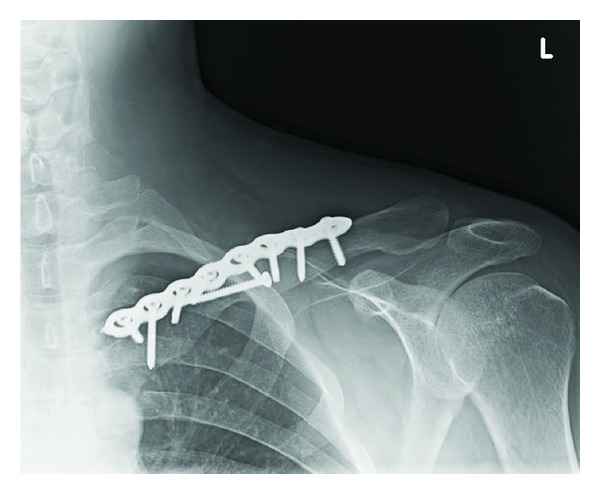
Radiographs taken after removing the medial one-third of the plate and four screws.
